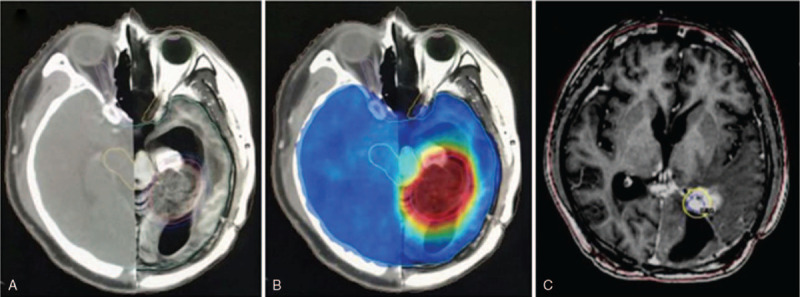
A: The clinical target volume and organs at risk were delineated in the CT-MR fusion images. B: Colorwash of dose distribution within the patient. The 95% of prescribed dose of blue area represents the treatment volume of whole-brain is 36 Gy, and the 95% of prescribed dose of red area represents the treatment volume of tumor is 58 Gy. C: SRS to the left lateral ventricle for the second stage. The yellow line represents the treatment volume, and the blue line represents the 13 Gy isodose line. SRS = stereotactic radiosurgery.
